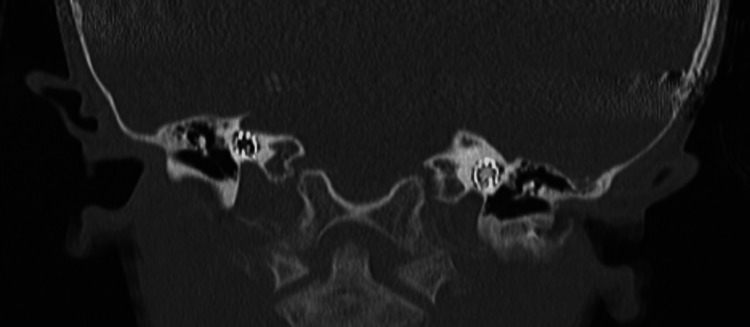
CT temporal bone coronal view showing left cochlear near complete ossification; the right cochlea is healthy-looking. Both cochlear implant electrodes are intact within the cochlea.
